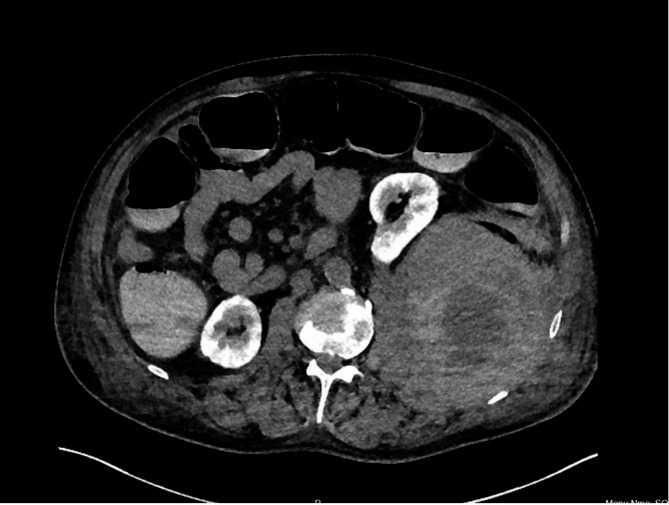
Computed tomography scan of abdomen showing retroperitoneal haematoma (red arrow) involving the left psoas muscle, with displacement of the left kidney (yellow arrow) anteriorly
